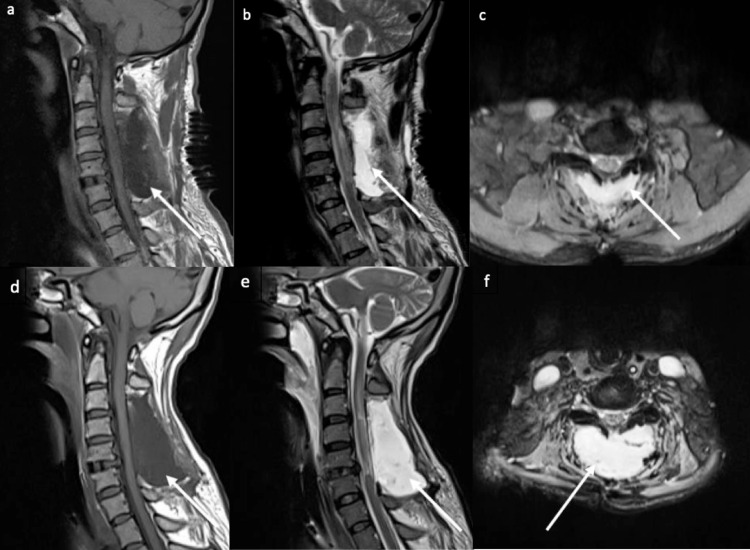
MRI of the cervical spine. Immediate post-surgical posterior decompression (a-c) demonstrates CSF-like signal collection representing a large pseudomeningocele (arrows) at the posterior para-spinal region. Follow-up cervical MRI images after two months (d-f) revealing interval increase in the size of the pseudomeningocele suggesting CSF leak. CSF - cerebrospinal fluid
